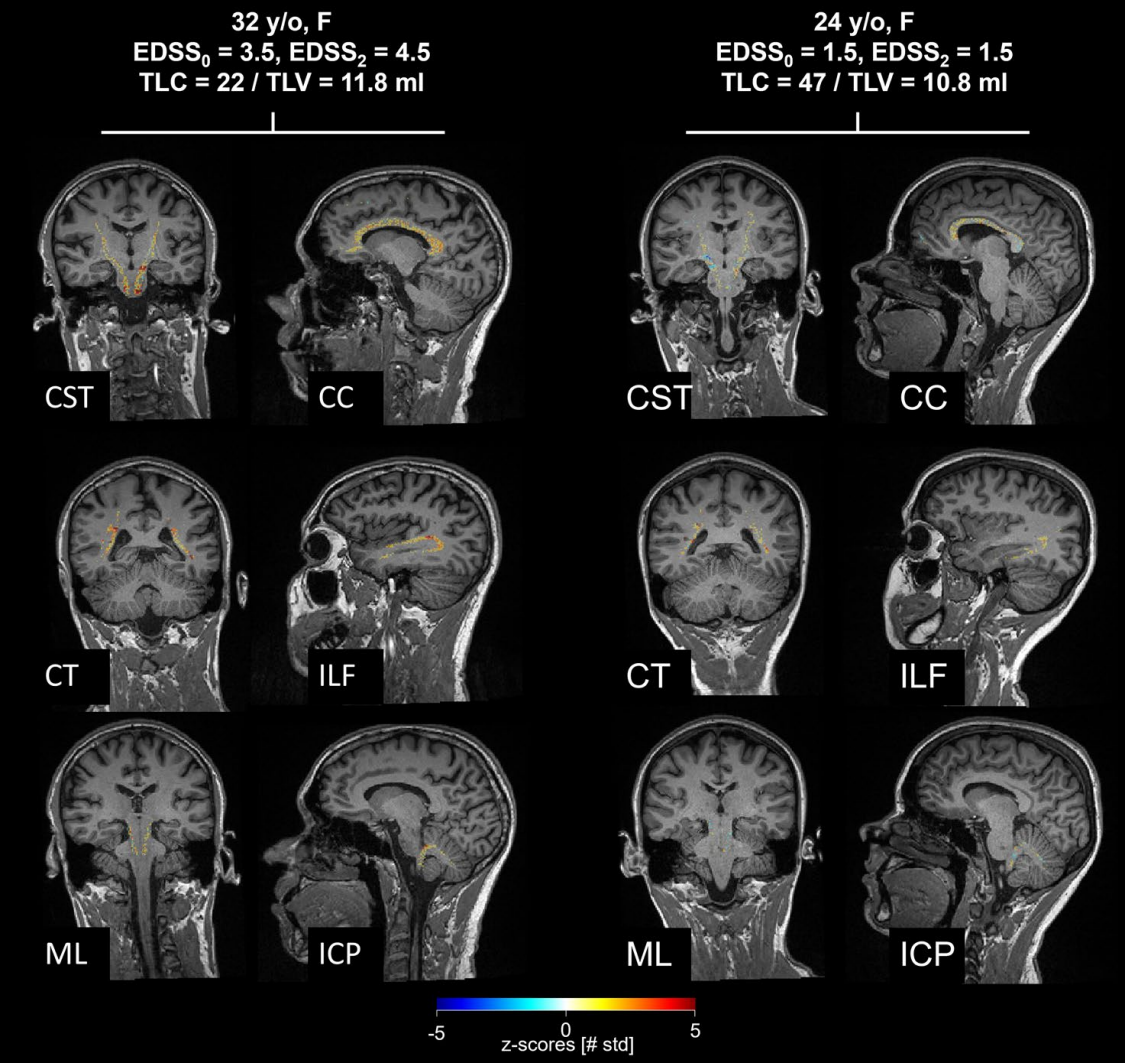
White matter tract-specific T1 *z*-scores shown in two example early MS patients. Despite similar total lesion load, the patient on the left, whose EDSS increased over 2 years, presented more severe alterations of the NAWM compared the patient on the right, who was clinically stable. *CST* cortico-spinal tract; *CT* corticostriatal pathway; *ML* medial lemniscus; *CC* corpus callosum; *ILF* inferior longitudinal fasciculus; *ICP* inferior cerebellar peduncle; *SST* spinothalamic tract; *TLC* total lesion count; *TLV* total lesion volume; *EDSS* expanded disability status scale
